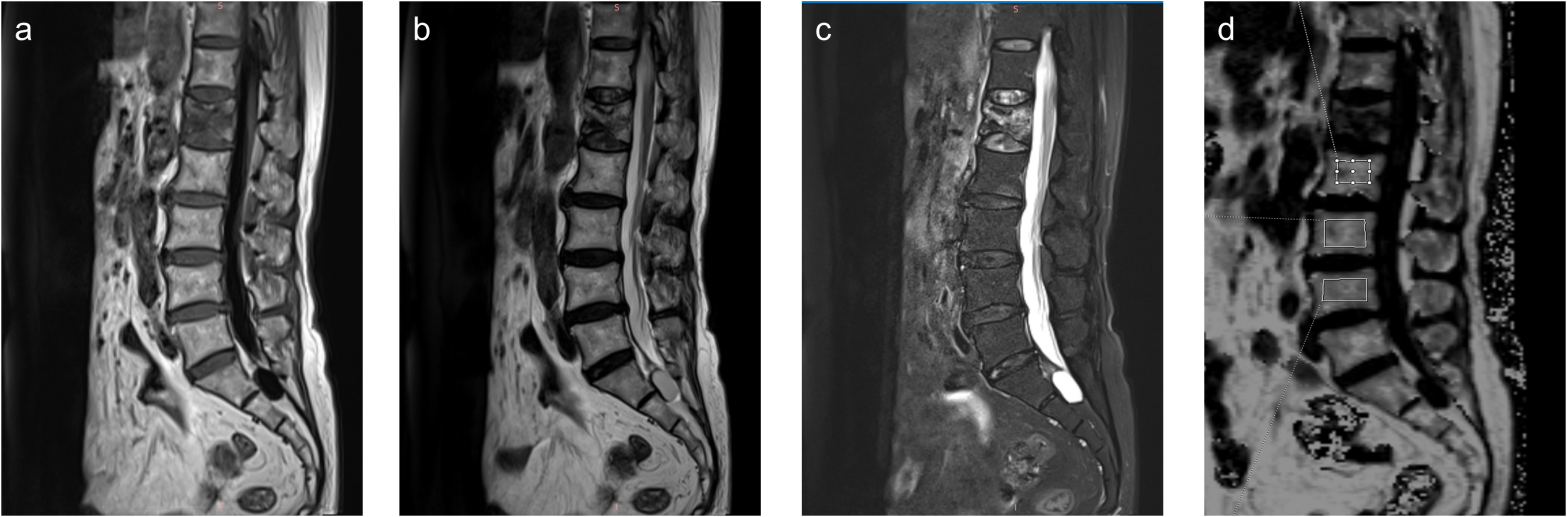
Sagittal T1-weighted (a), T2-weighted (b), Fat-sat T2-weighted (c) images and fat fraction (FF) map (d) of a 73-year-old female patient with an osteoporotic vertebral compression fracture in L1 vertebrae.
